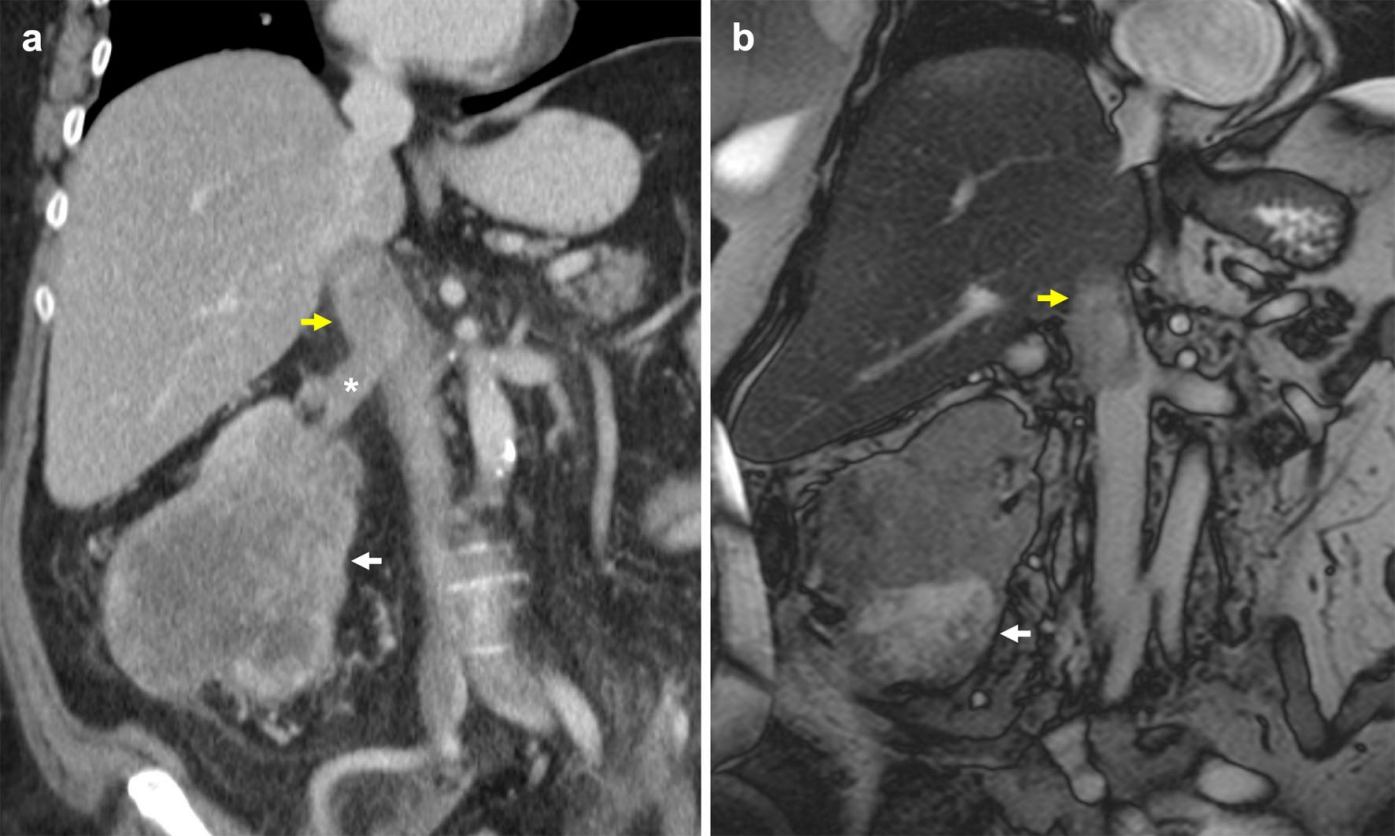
Renal cell carcinoma with Mayo level III thrombus. a Coronal CT shows a large heterogeneous renal mass (white arrow), expanded right renal vein (asterisk) and heterogeneous enhancement extending into the suprarenal IVC (yellow arrow) in keeping with tumour thrombus (b) T2-weighted coronal image confirms extension of tumour thrombus into the suprarenal and intrahepatic IVC (yellow arrow), which returns similar heterogeneous T2 signal as the primary renal cell carcinoma (white arrow)
